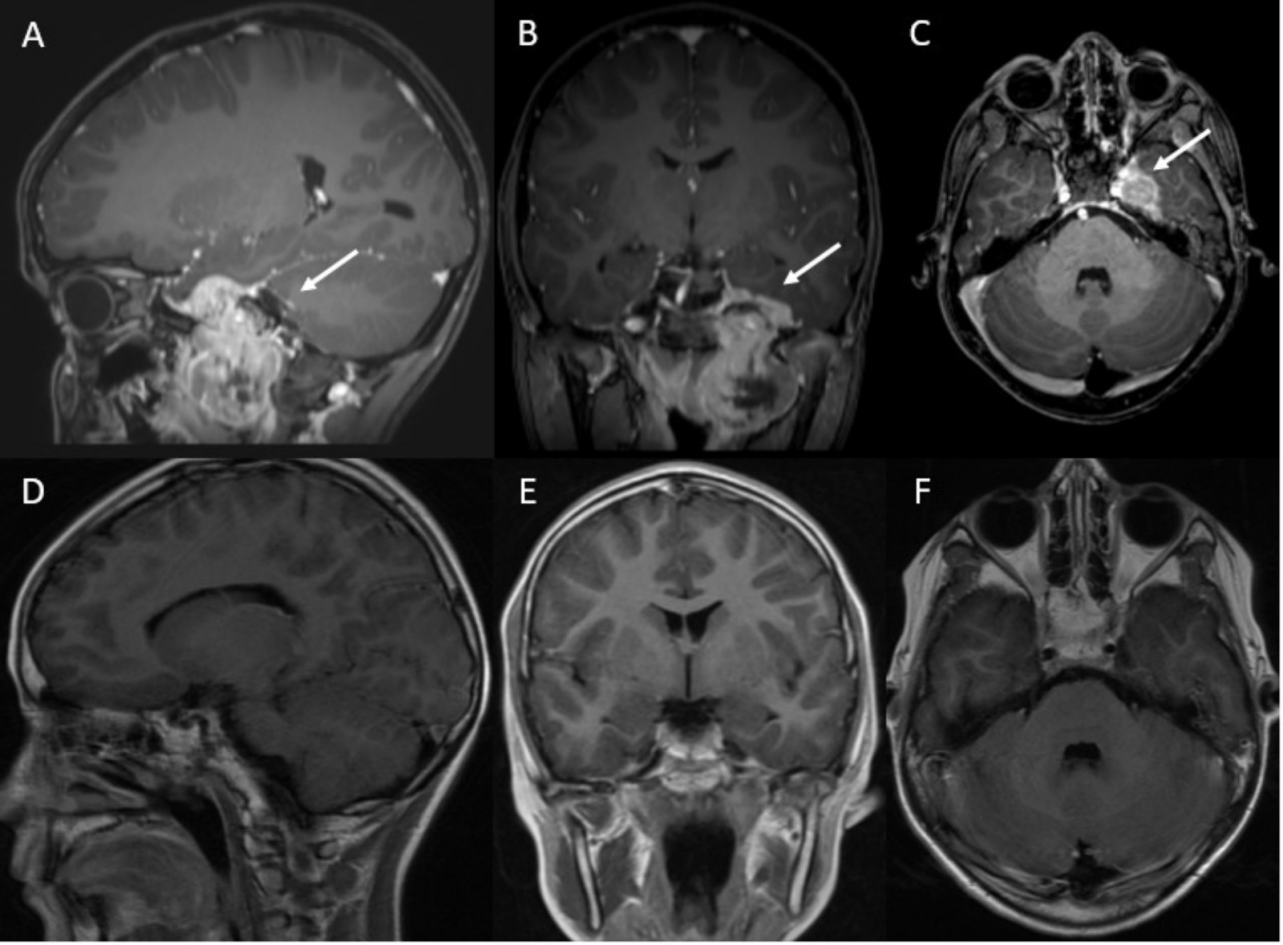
Parameningeal rhabdomyosarcoma in a 10-year-old male Sagittal (A), coronal (B), and axial (C) slices of T1-weighted MRI brain showing a hyperintense, enhancing lesion involving the left foramen ovale (blue arrows) pre-radiotherapy. Sagittal (D), coronal (E), and axial (F) slices of T1-weighted MRI brain showing no parenchymal signal abnormalities post-radiotherapy.
